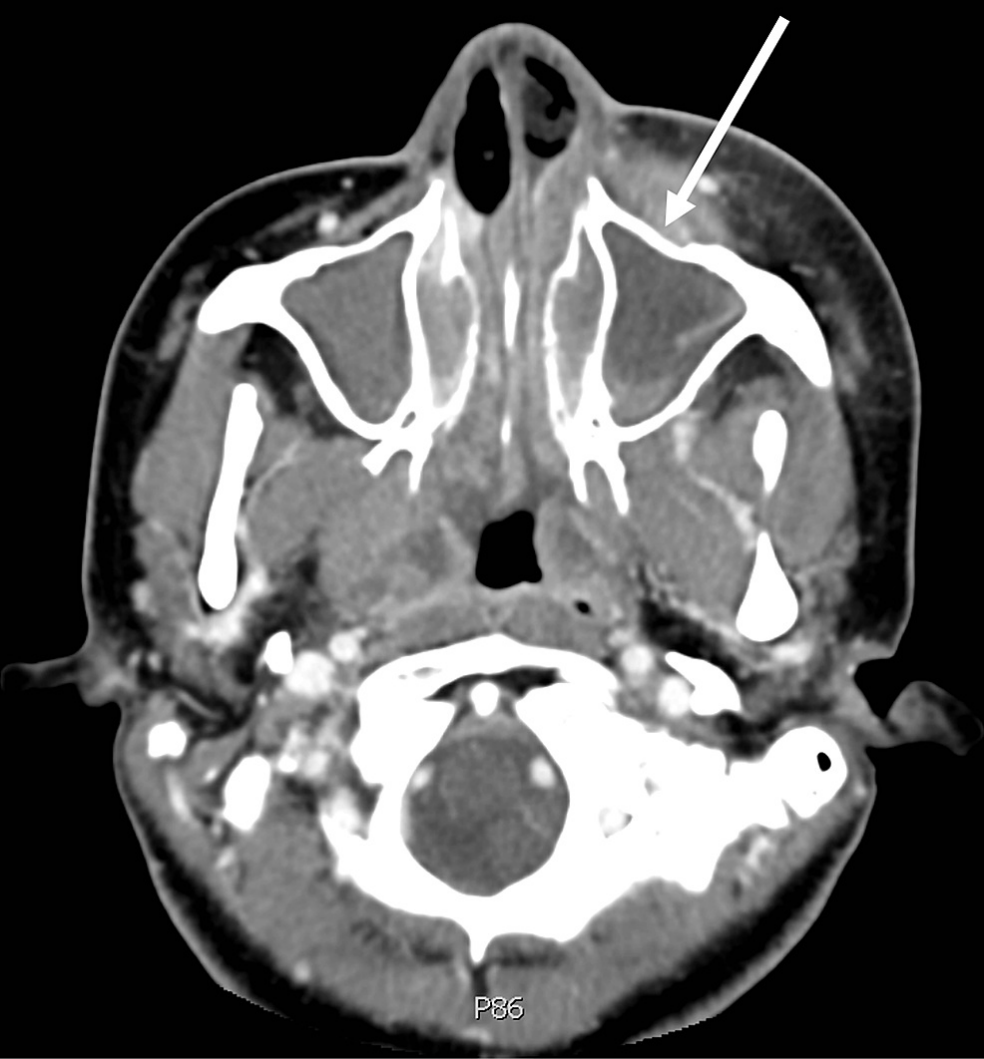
Axial slice of a CT with contrast of the sinuses demonstrating complete opacification of parasinuses and nasal cavity with aggressive osseous changes (white arrow) and diffuse fat stranding with concern for invasive sinusitis with pre- and post-septal orbital tissue involvement.
